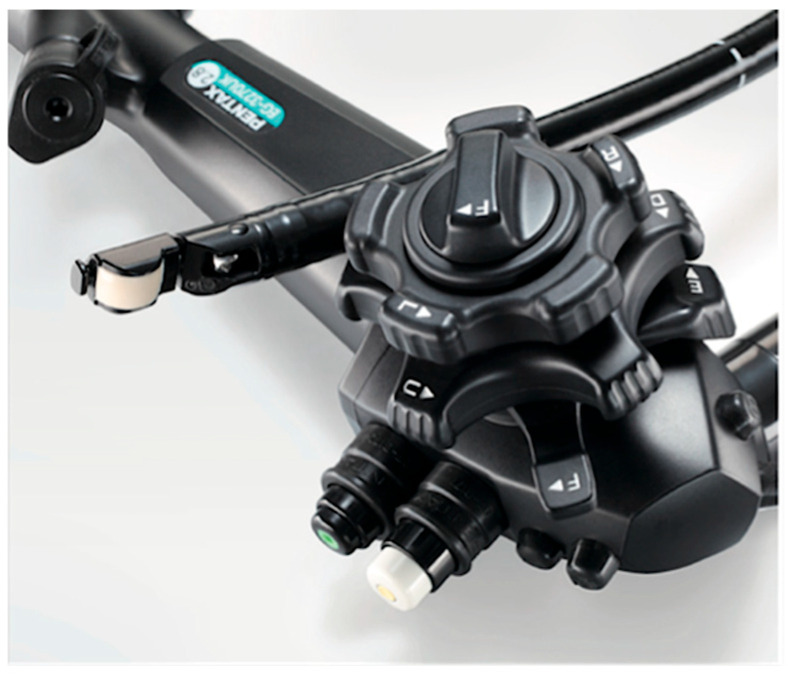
The main features of endoscopic ultrasound EG-3270UK, (Hitachi Medical Systems Europe, Sumpfstraße 13, 6300 Zug, Switzerland, now belonging to Fujifilm), at the proximal and distal ends of the probe [11].
